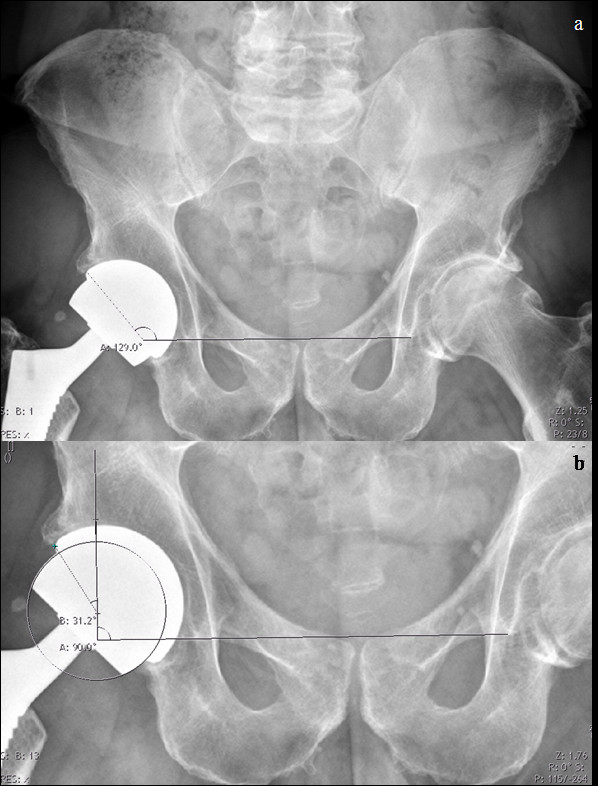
a&b. Plain radiographs of the pelvis of a 46-year-old female patient with an ASR^TM^XL Head device two years following implantation.a) Measuring cup inclination. b) Measurements to determine the arc of cover according to the method described by De Haan et al. in 2008.
